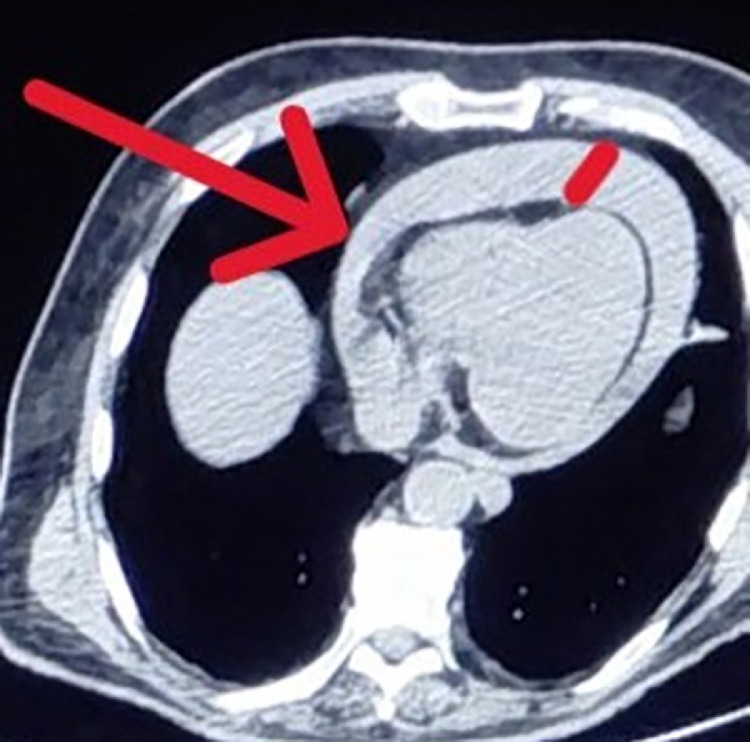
Emergency axial CT performed immediately after hemodynamic collapse. The red arrow points to the large hemopericardium and right heart diastolic collapse (cardiac tamponade).•Emergency US-guided pericardiocentesis was performed at the bedside. Approximately 160 mL of hemorrhagic fluid was manually aspirated, leading to immediate normalization of vital signs. An 8F pigtail catheter was placed for continuous drainage (Fig. 3B). Post-procedural hemoglobin levels showed a minor drop (from 11.8 g/dL to 10.4 g/dL), which remained stable without transfusion. The patient was discharged after 5 days. A one-month follow-up MRI showed complete technical success with a non-enhancing ablation zone and total resolution of the hemopericardium (Fig. 4).
